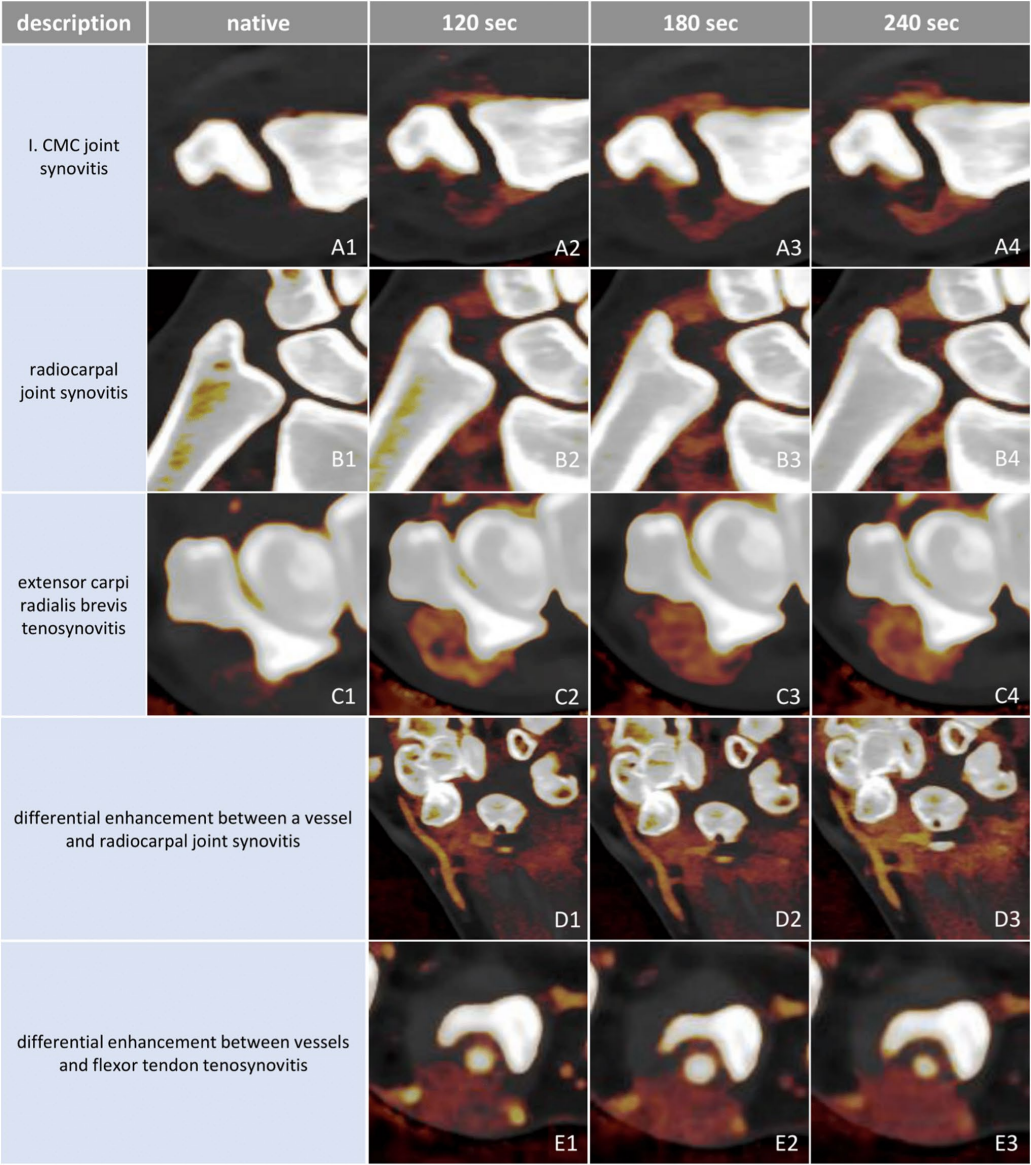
Appearances of enhancing inflamed synovial and tenosynovial structures, as well as contrast in enhancement between vessels and inflamed tissues across native and investigated post-contrast phases seen on PCD-CT iodine maps. In the native phase, inflamed synovial and tenosynovial structures show no enhancement (A1, B1, C1). As for the post-contrast phases, they display very similar enhancement intensities and spatial distributions of inflammation across each phase. The apparent differences in enhancement observed between the 240-s phases and the earlier ones are due to the normalization of the iodine map’s color coding to an enhancing vessel, which gradually washes out. This effect can be corrected by manually adjusting the windowing, enabling more or less color to be attributed to the same iodine concentration values. The greatest contrast between enhancing vessels and inflamed tissues was seen in the 120-s phase, as demonstrated by rows D and E. CMC, carpometacarpal; PCD-CT, photon-counting detector CT; sec, second
